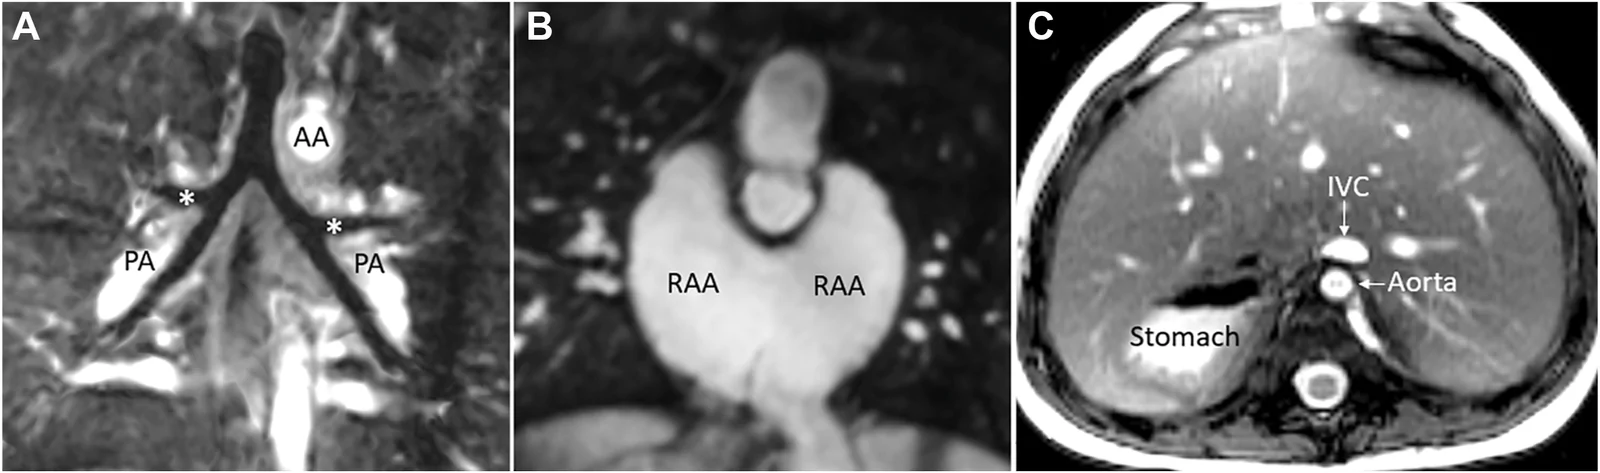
Magnetic resonance images from a patient with heterotaxy, right isomerism of the atrial appendages, and asplenia. (A) The bronchial length is asymmetric with a ratio of 2.02. However, the upper lobar bronchus is above the descending branch of the ipsilateral pulmonary artery (PA) on both sides. (B) The appendages of both atria are triangular with a wide orifice, which is characteristic of the morphologically right atrial appendage (RAA). (C) There is no spleen behind the stomach. The inferior vena cava (IVC) and abdominal aorta are juxtaposed on the left side of the spine. AA, aortic arch.
